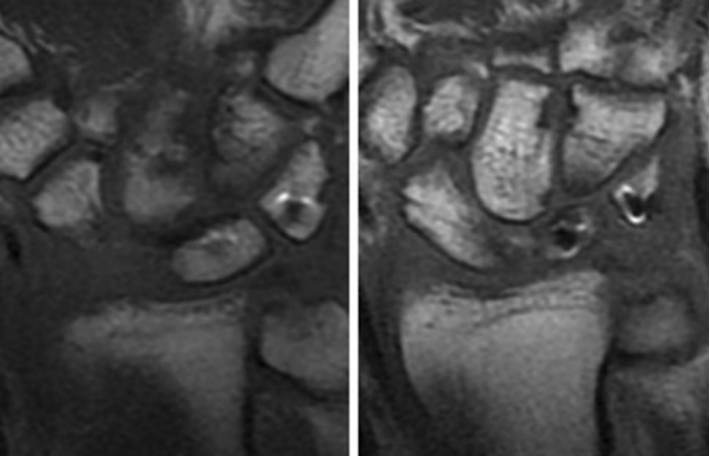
Magnetic resonance imaging at the 1-year follow-up showing no evidence of avascular necrosis of the scaphoid and lunate
